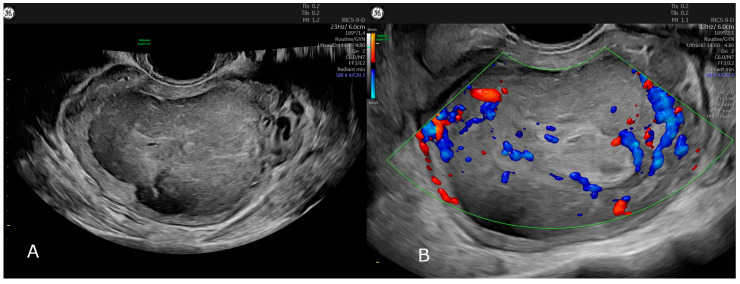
(A) Grayscale transvaginal ultrasound of the index case. A 53 × 39 × 58 mm heterogeneous myometrial mass is identified at the uterine fundus, classified FIGO type 2–5. The lesion displays irregular multilobulated margins, mixed isoechoic and hyperechoic internal texture, extension to the fundal serosa (residual myometrial margin < 1 mm), and complete absence of posterior acoustic shadowing. No calcifications are visible. (B) Color Doppler transvaginal ultrasound of the same lesion. A Color Score (CS) of 3 is documented, reflecting moderate intralesional vascularization.
